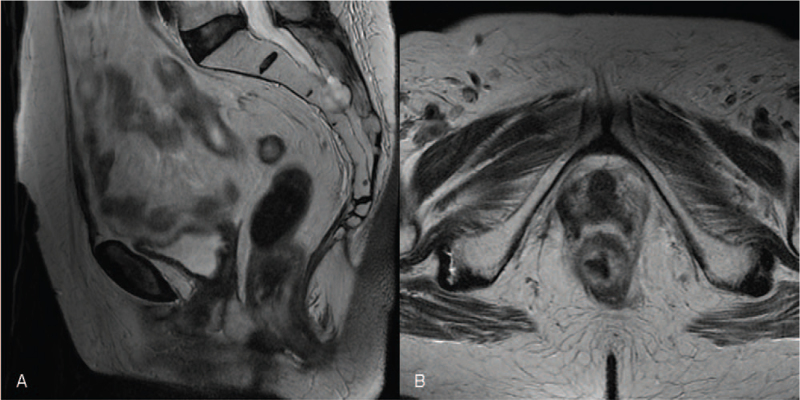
Sagittal (A) and axial (B) T2-weighted magnetic resonance images performed 45 days after treatment. No signs of previous-seen mass-like thickening of the rectal and anal canal wall on T2-weighted images.
